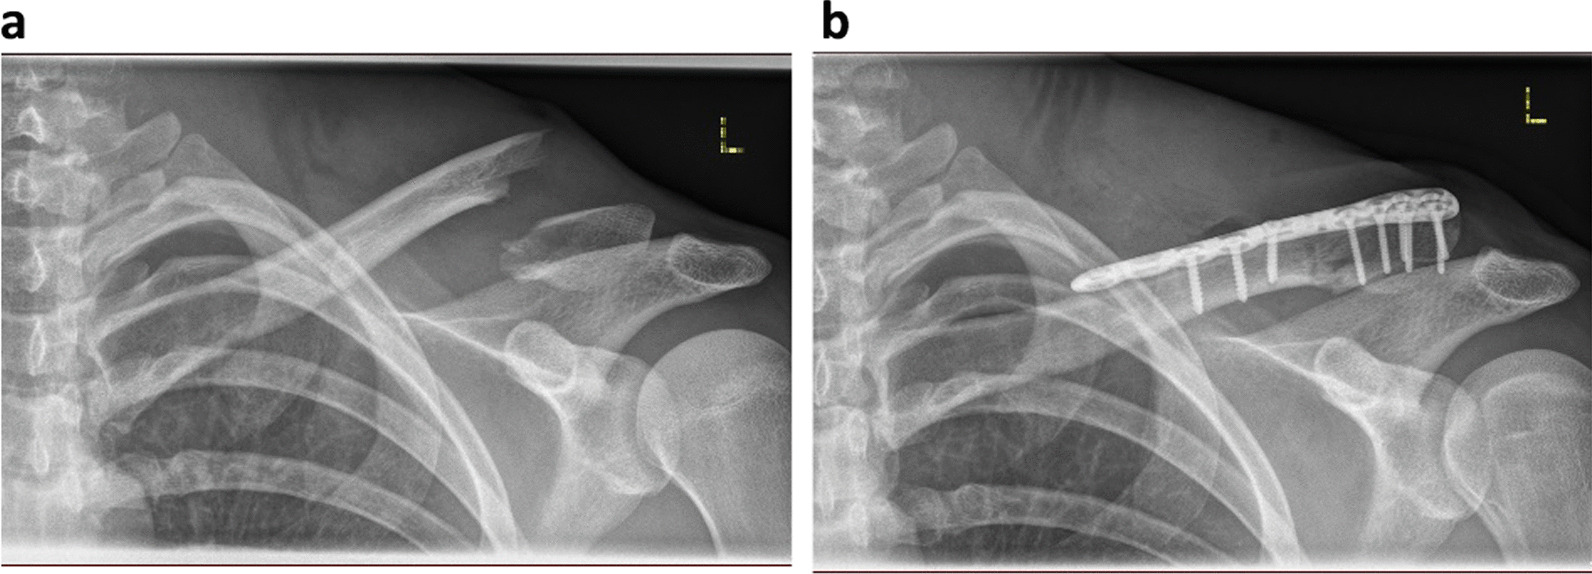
a Radiograph showing left clavicle fracture Typ IIa after the bike accident. b Post-surgery radiograph showing left clavicle plate system (VariAx) after plate osteosynthesis
